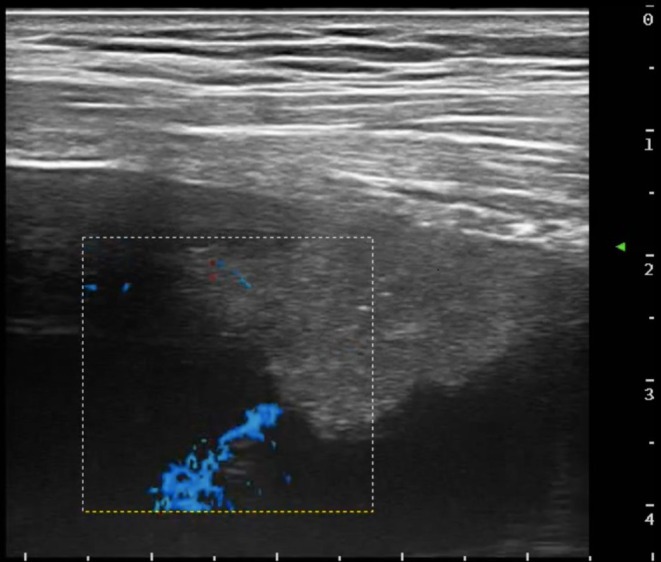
Colour Doppler ultrasound findings suggestive of active intrapleural bleeding from the biopsy site.
